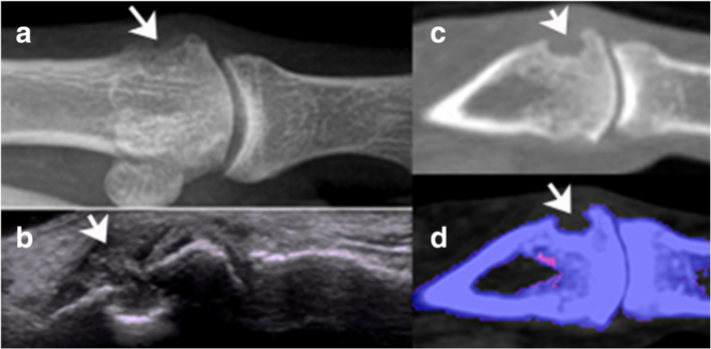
Patient 14. Erosion of distal right metatarsal head, imaged by radiograph (panel (a), arrow), ultrasound (panel (b), arrow), CT (panel (c), arrow) and DECT (panel (d), arrow). Fluffy, hyperechoic material is evident in the synovial space on ultrasound (panel (b), arrow). Note the absence of any green pixellated areas (MSU deposits) in panel (d). The DECT scan was positive for MSU deposits in the left distal metatarsal head (not shown)
